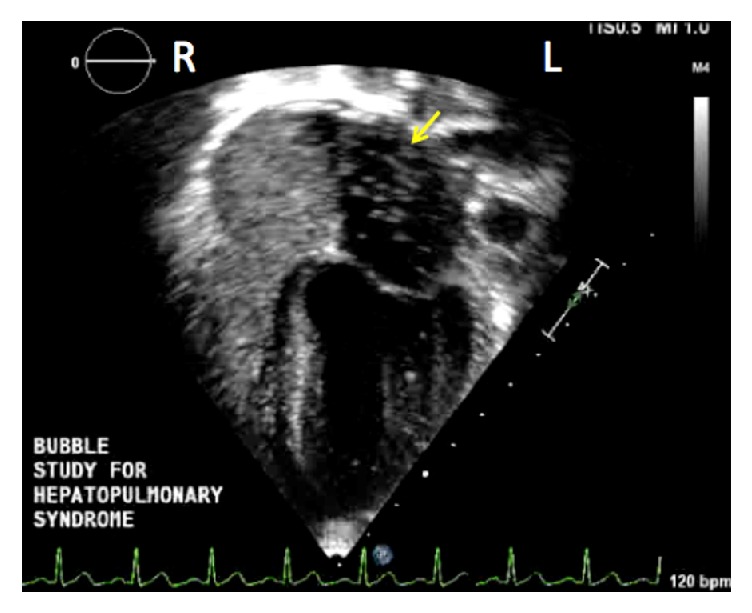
Two-dimensional contrast echocardiography showed the presence of bubbles in the left atrium after 7 cardiac cycles (yellow arrow), consistent with an intrapulmonary shunting (R: right, L: left).
